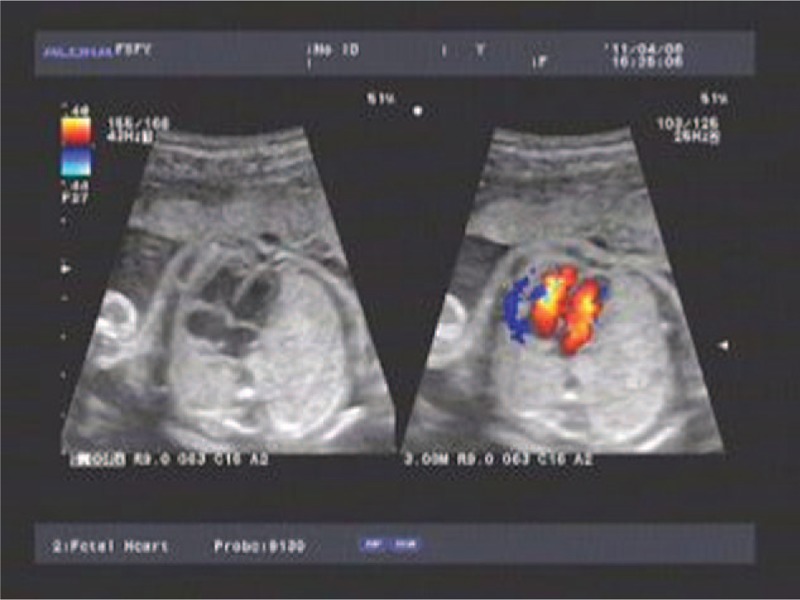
Sonographic measurement of the heart function.
